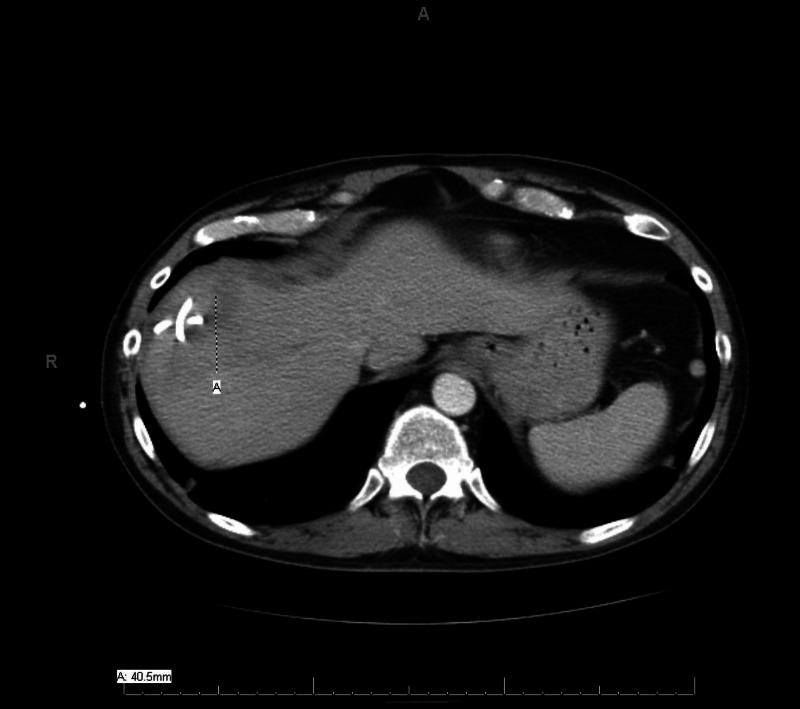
Repeat CT scan of abdomen and pelvis The cross mark indicates a significant decrease in the liver abscess size to 4.1 x 4.3 cm (repeat CT performed four weeks after the rehab treatment) CT: computed tomography
